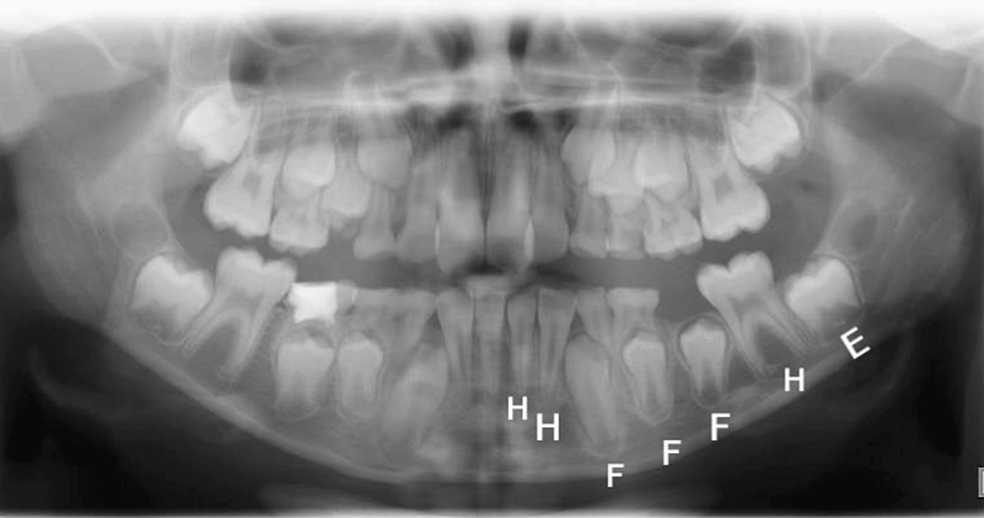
Panoramic radiograph with stages of calcification from E to H
